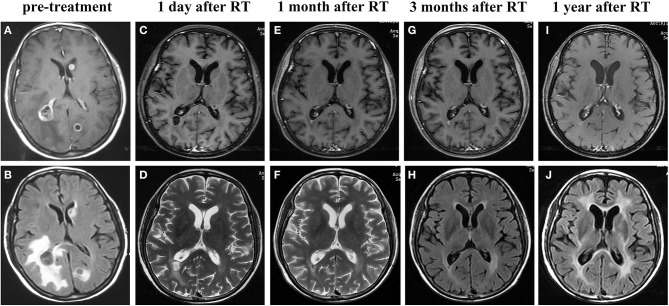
Representative magnetic resonance imaging images of the brain metastatic lesions at different time points. Prior to the treatment showing lesions in the left occipital lobe, right temporo-occipital lobe junction and a large region of edema according to enhanced T1-weighted MRI (A) and T2-weighted FLAIR MRI (B). On the first day after finishing the whole course of brain radiotherapy, showing shrinkage of tumors in enhanced T1-weighted MRI (C) and T2-weighted MRI (D), along with marked alleviation of cerebral edema. Enhanced T1-weighted MRI (E,G,I),T2-weighted MRI (F) and T2-weighted FLAIR MRI (H,J) performed at 1, 3, 12 months after brain radiotherapy showed the brain tumors were well controlled. RT, radiotherapy.
